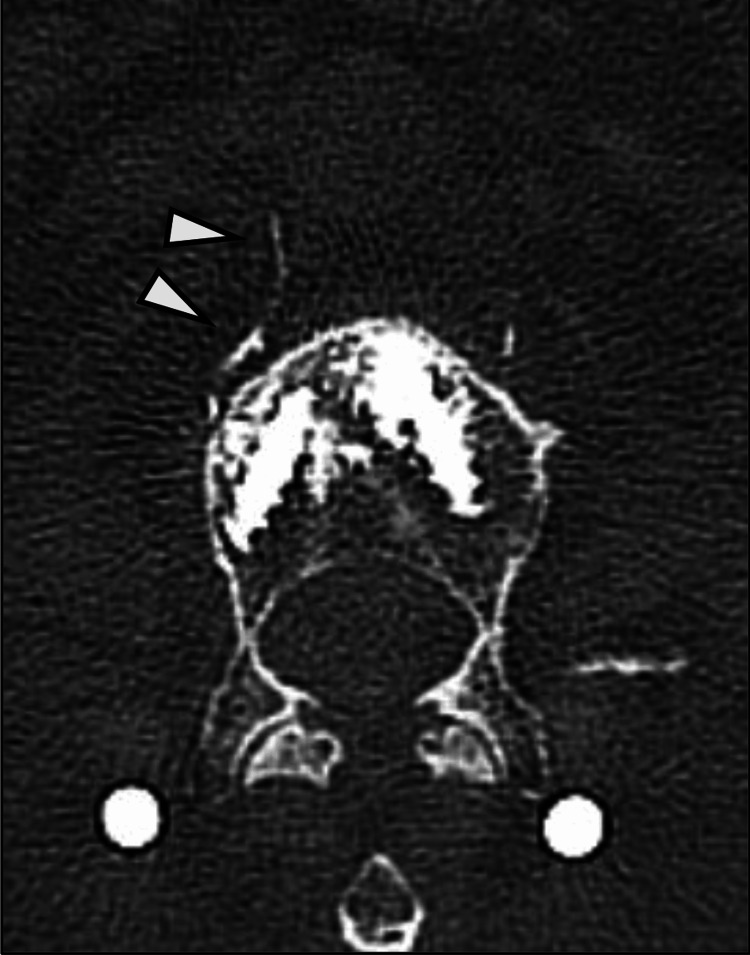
CT image showing cement leakage into the inferior vena cava CT: computed tomography
